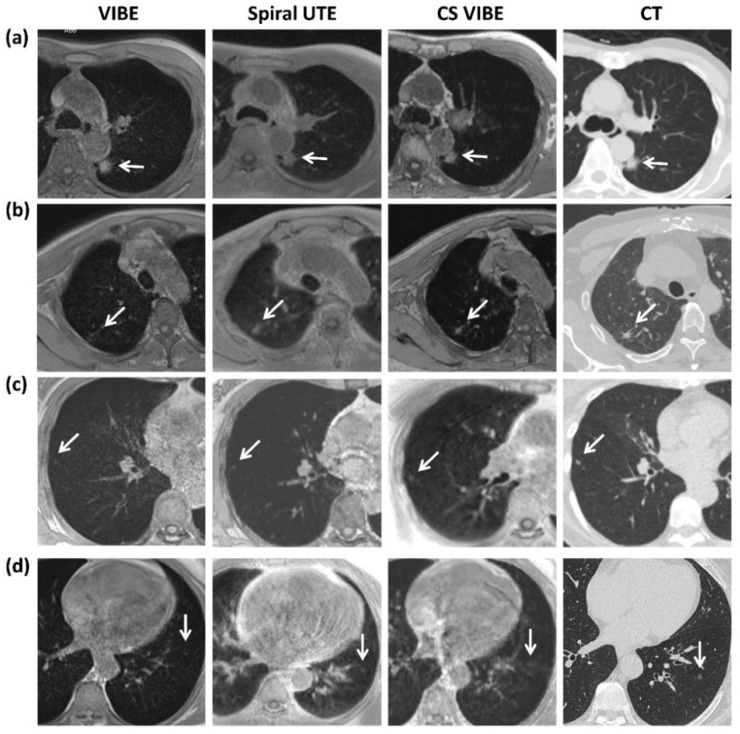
(a) Example of a 63-year-old man with an 18 mm part-solid nodule (white arrow) in the left lower lung shown on a reference axial CT image, detected clearly on conventional breath-hold VIBE, free-breathing spiral UTE MR, and breath-hold CS-VIBE images. (b) Example of a 66-year-old man with a 6 mm solid nodule (white arrow) in the right upper lung shown on a reference axial CT image, blurred on conventional breath-hold VIBE, and clearly depicted on free-breathing spiral UTE MR and breath-hold CS-VIBE images. (c) Example of a 55-year-old woman with a 4 mm solid nodule (white arrow) in the right lower lung shown on a reference axial CT image, detected vaguely on conventional breath-hold VIBE, and clearly depicted on free-breathing spiral UTE MR and breath-hold CS-VIBE images. (d) Example of a 60-year-old woman with a 4 mm non-solid nodule (white arrow) in the left lower lung shown on a reference axial CT image, detected on free-breathing spiral UTE, which could be easily missed on conventional breath-hold VIBE or CS-VIBE images due to cardiac pulsation artifacts. Abbreviations: MR: magnetic resonance; VIBE: volumetric interpolated breath-hold examination; UTE: ultrashort echo time; CS: compressed sensing.
